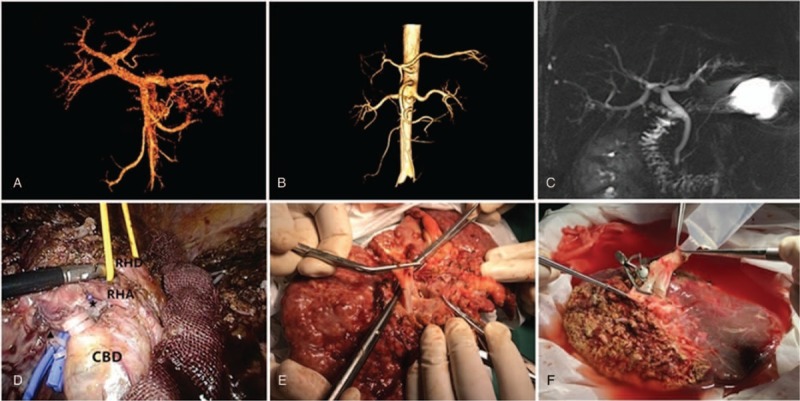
(A) Anatomy of donor's portal vein by CT scan. (B) Anatomy of donor's hepatic artery by CT scan. (C) Anatomy of donor's biliary tree by MRCP. (D) Dissection of portal veins (★: right anterior portal vein; ▴: right posterior portal vein), hepatic artery and bile duct. RHA: right hepatic artery; RHD: right hepatic duct; CBD: common bile duct. (E) The Y-graft of the recipient's own portal confluence. (F) Right liver graft with reconstruction of separate right anterior and posterior portal veins using a Y-graft of the recipient's own portal confluence.
